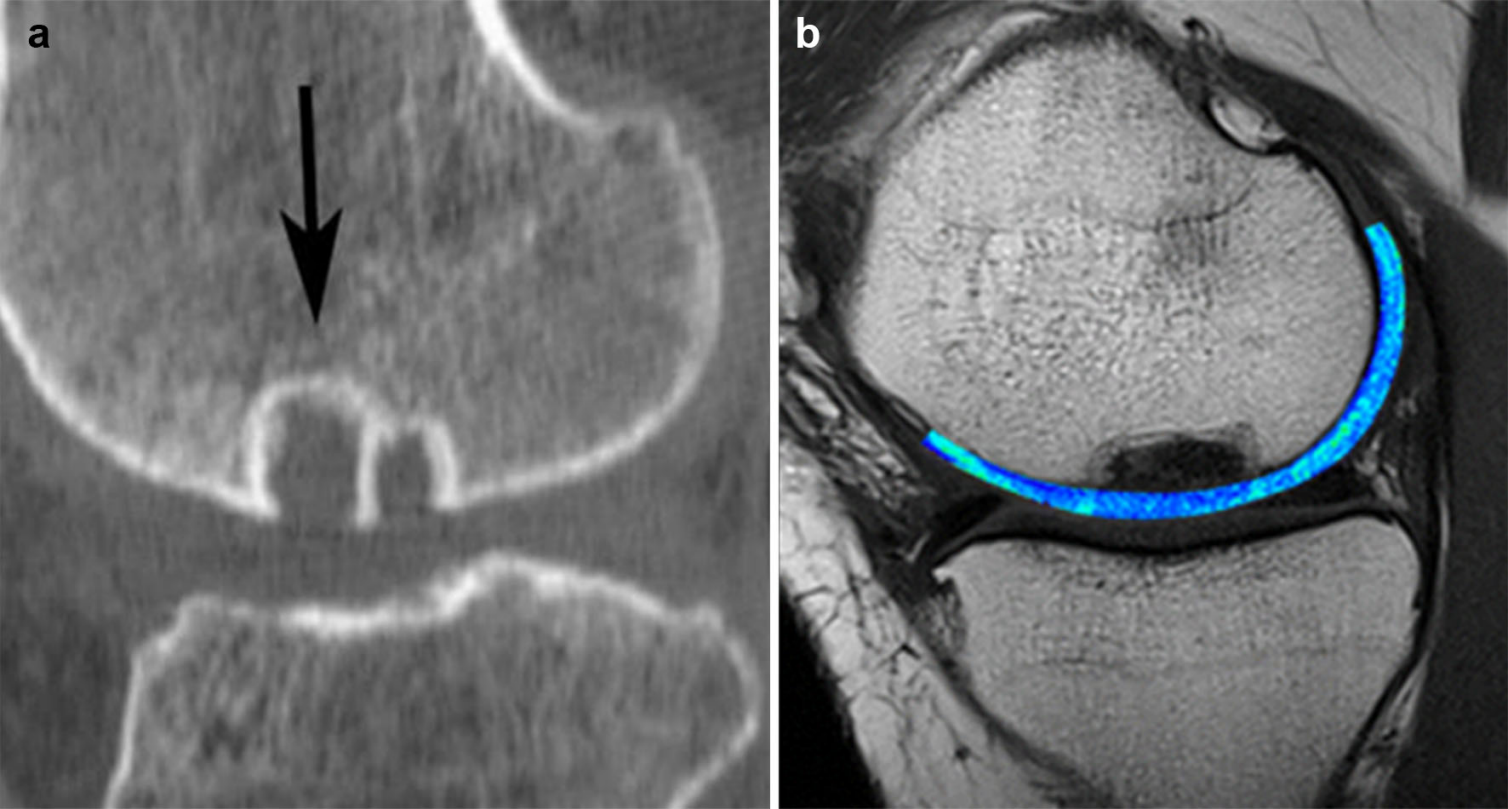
a CT scan failed to show bone ingrowth (*arrow*) into TruFit plug [90]; b T2 mapping MRI scan of OC lesion repair after 18 months by the use of the MaioRegen$$\circledR $$ scaffold, and bone cysts are observed [96]. With permissions from Elsevier and Springer
